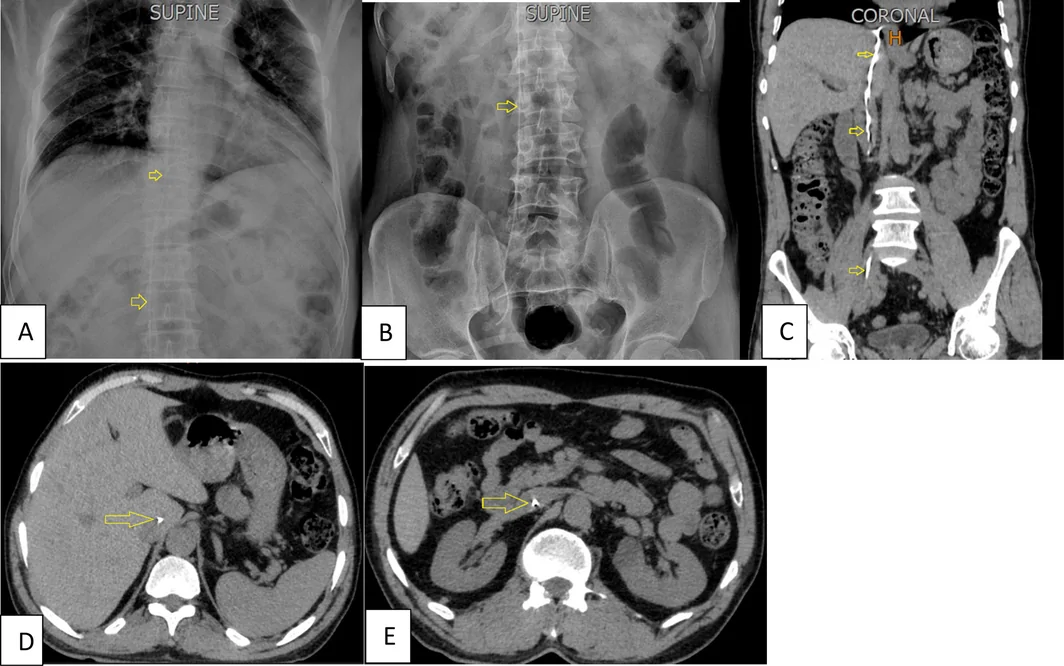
(A, B) The double‐J catheter has migrated into the IVC, as demonstrated on radiographs. The catheter tip is visualized in the right atrium. (C–E) The double‐J catheter was shown in coronal and axial views of a CT scan.
